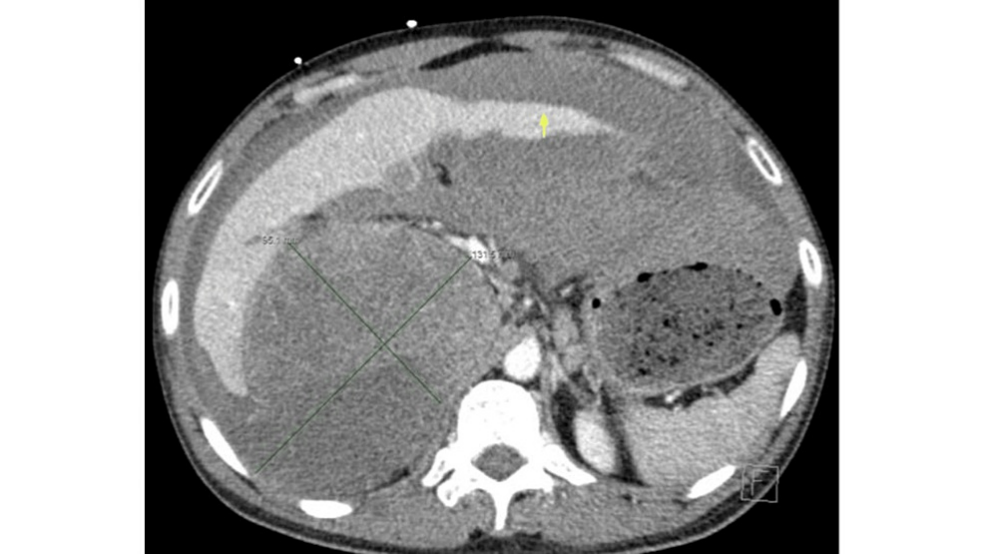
Axial view of the CT demonstrates a large right abdominal heterogeneous enhancing mass measuring 9.5 cm x 13.1 cm in length and width. The yellow arrow points to the hemoperitoneum.
